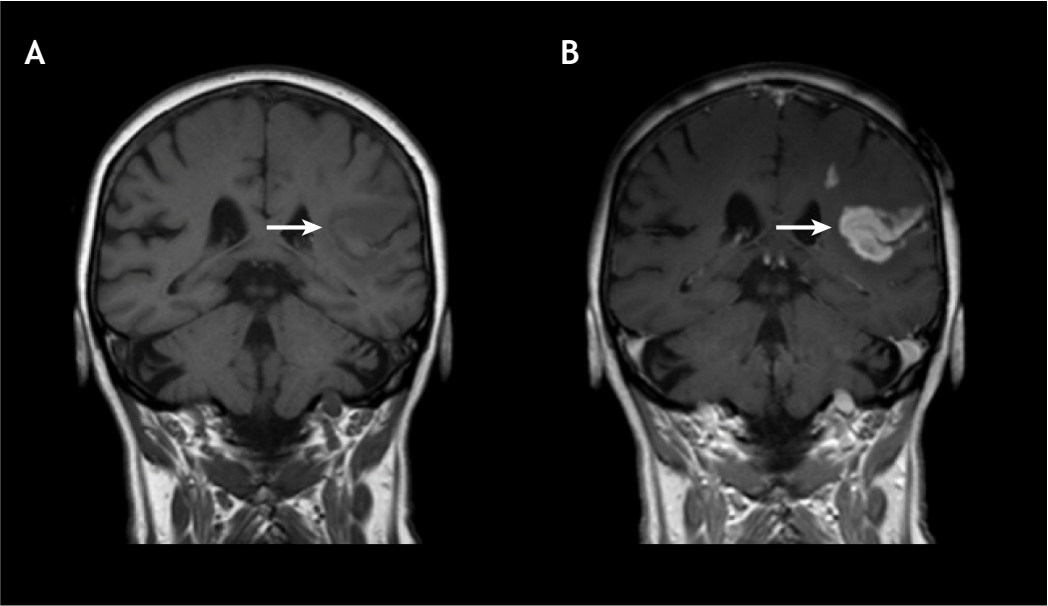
Effect of contrast agent. (A,B) T1-weighted MRI of an adult brain showing infarction (white arrows) without contrast agent (A) and with contrast agent (B). Images reproduced with permission from Friebe (2016). These images are not published under the terms of the CC-BY license of this article. For permission to reuse, please see Friebe (2016).
